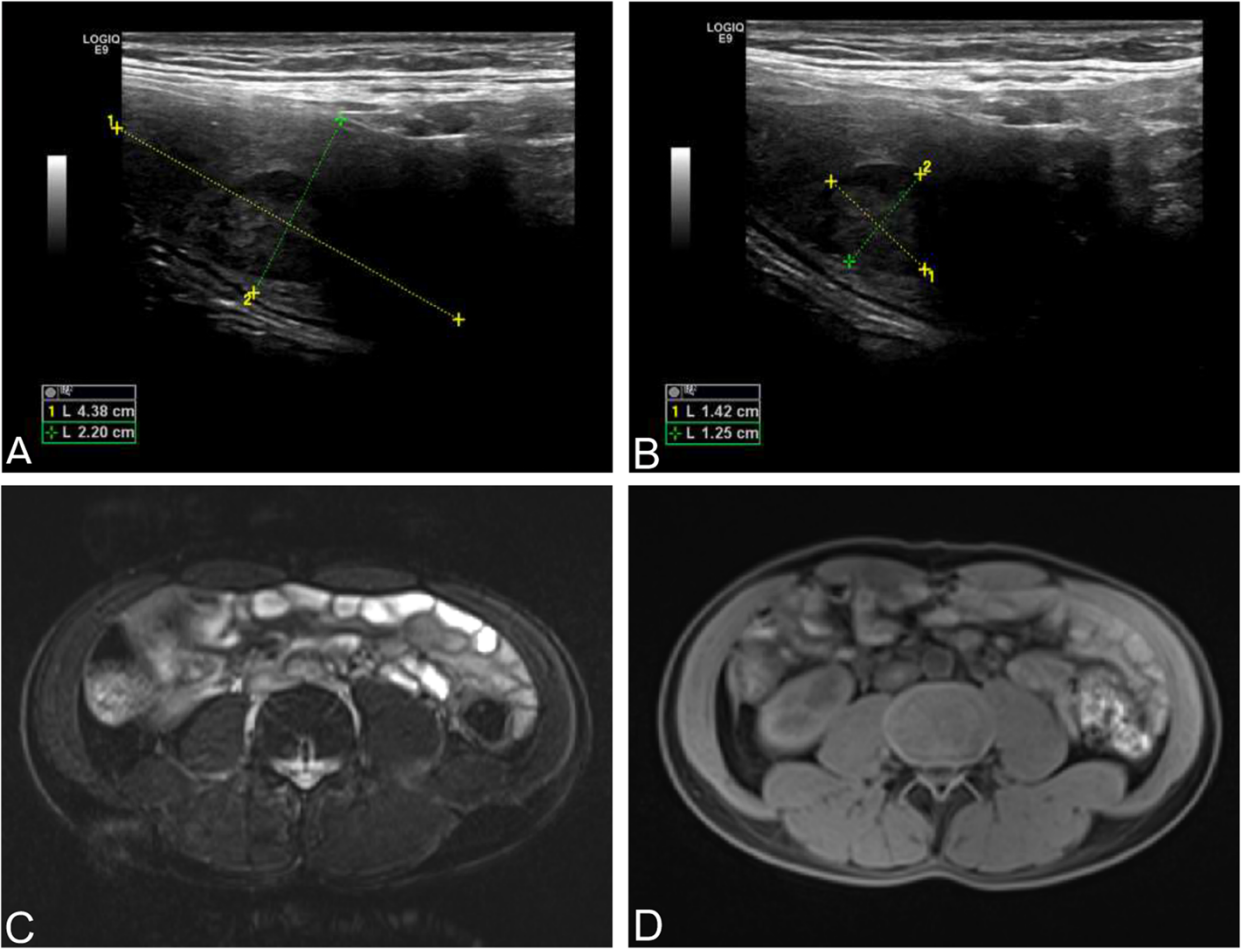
Imageological examination. a, b Abdominal ultrasonography showed the right undescended testicle in the abdomen, whose size was about 4.4 × 2.2 cm (a). Two regular and well-defined hypoechoic nodules were found in the right testicle with the larger one of about 1.42 × 1.25 cm (b). c, d The images of abdominal Magnetic Resonance Imaging (MRI)
